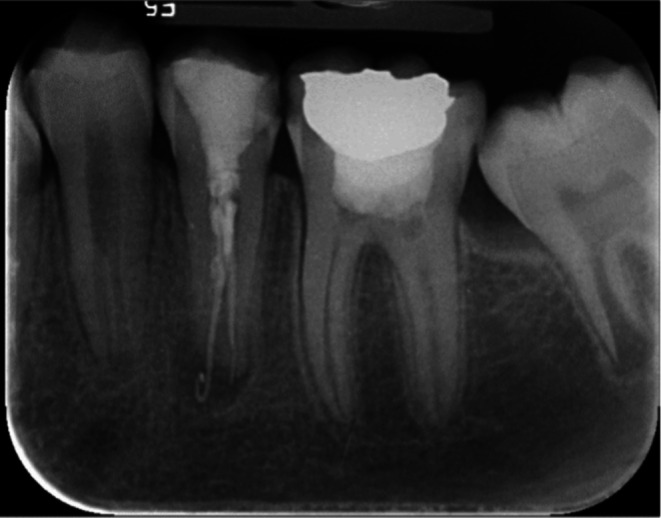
Initial Radiograph with poor‐quality previous root canal filling and periapical lesion.
